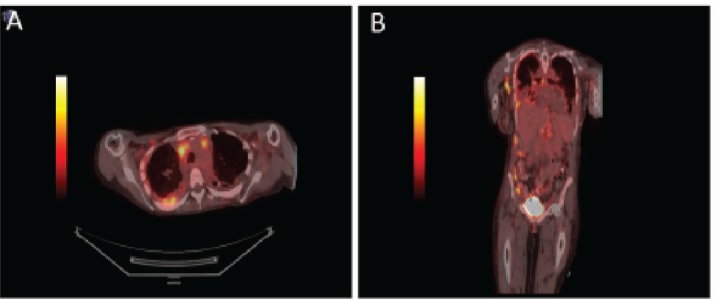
PET/CT body showing diffuse enhancement of disease. Axial views of the body on PET/CT demonstrating (a) intrathoracic lymphadenopathy and FDG avid right pleural thickening. Coronal view of demonstrating axillary lymphadenopathy, intrathoracic lymphadenopathy, right pleural thickening, FDG-avid caking on ascending colon and right lateral abdominal wall implant (b).
